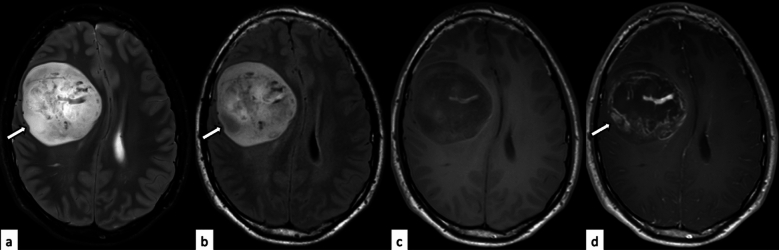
Diffuse astrocytoma, MYB-altered in an 18-year-old man. The tumor mass with well-circumscribed margin in the right frontal lobe shows hyperintensity on fat-saturated T2-weighted imaging (a) and fluid-attenuated inversion recovery (FLAIR) imaging (b), heterogeneous hypointensity on T1-weighted imaging (c), and heterogeneous contrast enhancement on contrast-enhanced T1-weighted imaging (d). Partial T2-FLAIR mismatch sign (a, b, arrows) is observed within the contrast-enhanced component (d, arrow), indicating that it is not a cystic degeneration. The same case with different images has been evaluated in our previous study [2]
